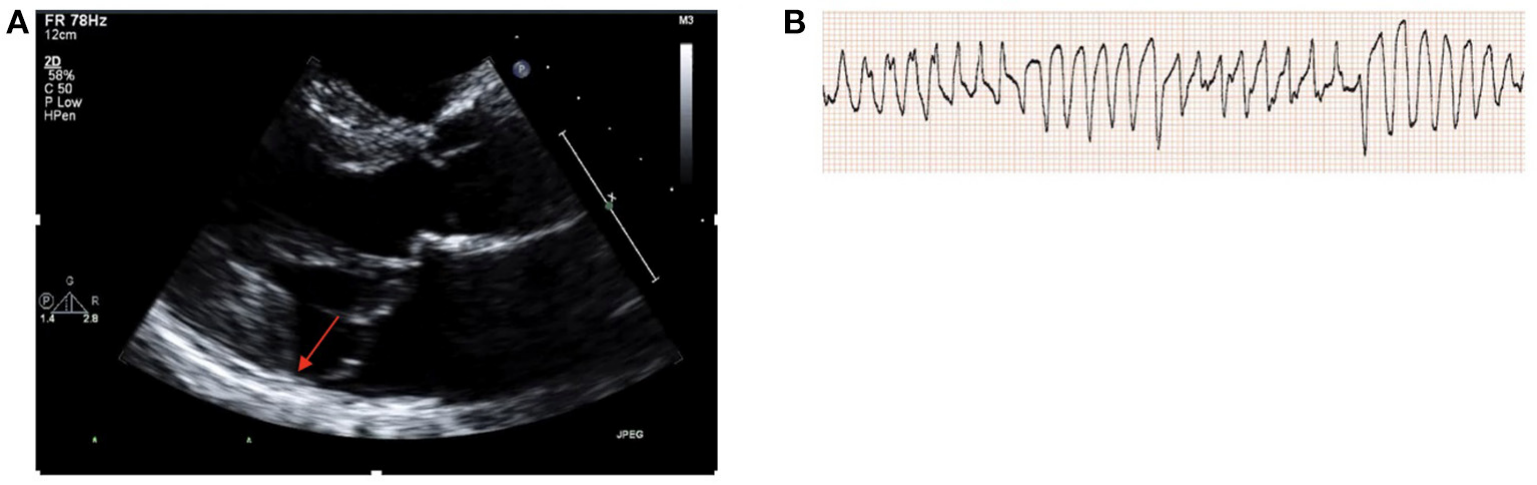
(A) TTE parasternal long axis demonstrating bileaflet MV prolapse and MAD (red arrow). (B) Induction of polymorphic ventricular tachycardia during electrophysiology study.
